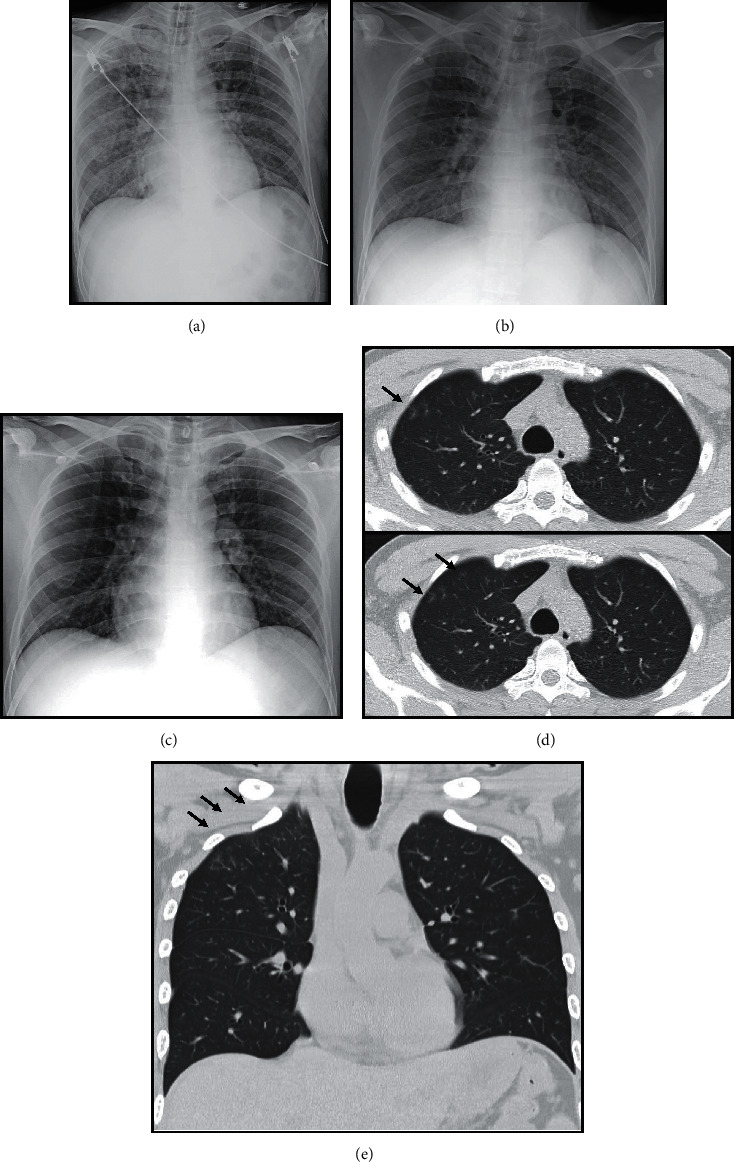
Images in a 35-year-old male with acute myeloid leukemia who had positive chest symptoms and serum galactomannan test. (A) Baseline chest film shows opacities at right upper and right lower lungs. (B) Chest film on second day of febrile neutropenia (ANC = 0 *μ*L) shows improvement of previous opacities with no new opacity. (C) Chest film on fifth day of febrile neutropenia (ANC = 0 *μ*L) shows no abnormal opacity. (D–E) Axial and coronal CT chest on seventh day of febrile neutropenia (ANC = 0 *μ*L) reveals subtle ground-glass attenuation at right upper lobe (arrows). This case was evaluated as false-negative chest radiograph and true-positive CT chest.
